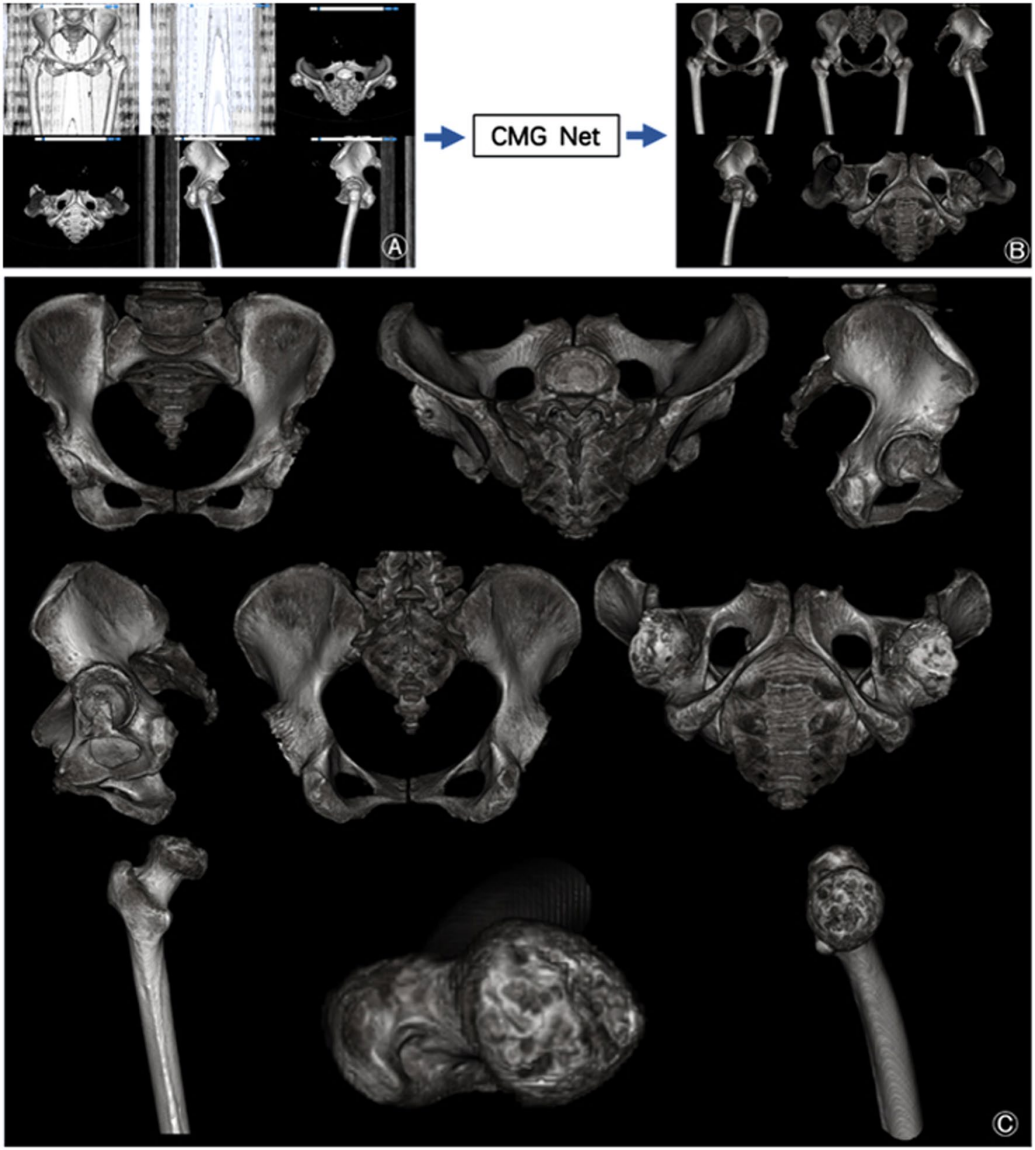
The CMG Net can accurately segment diseased hips. A 3D model rebuilt by original CT images; B 3D model rebuilt by CT images segmented by CMG Net; C the osteophytes and defects of both femur and acetabular can be observed clearly
